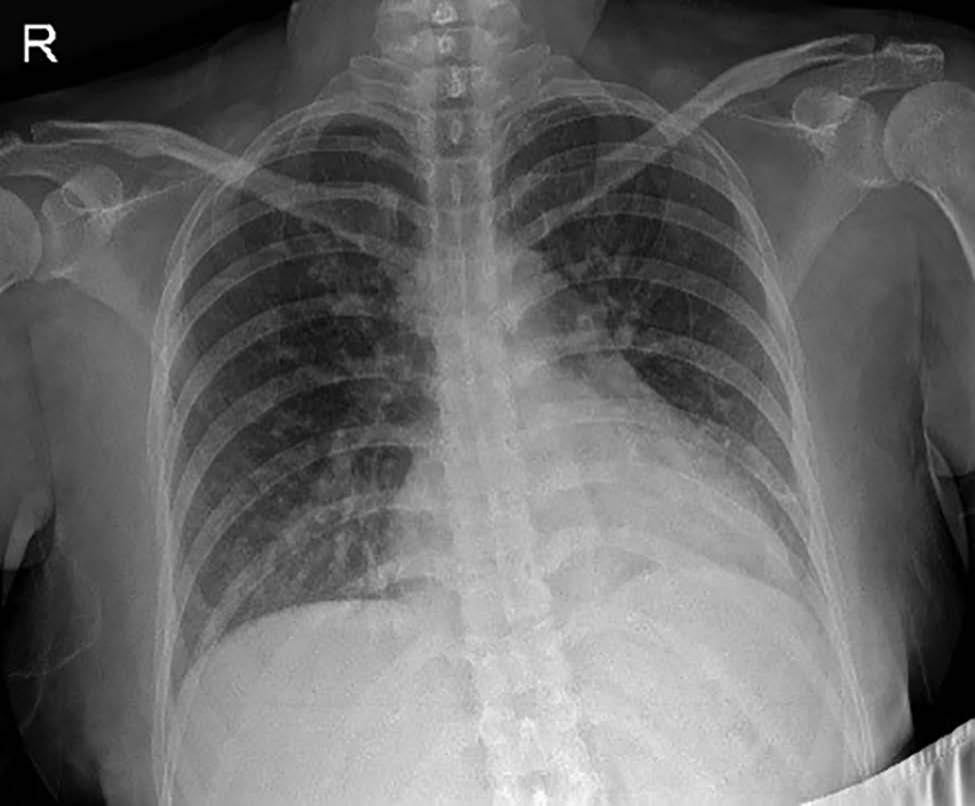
Chest X-ray at GA 30 + 5 weeks increased BLL haziness.
